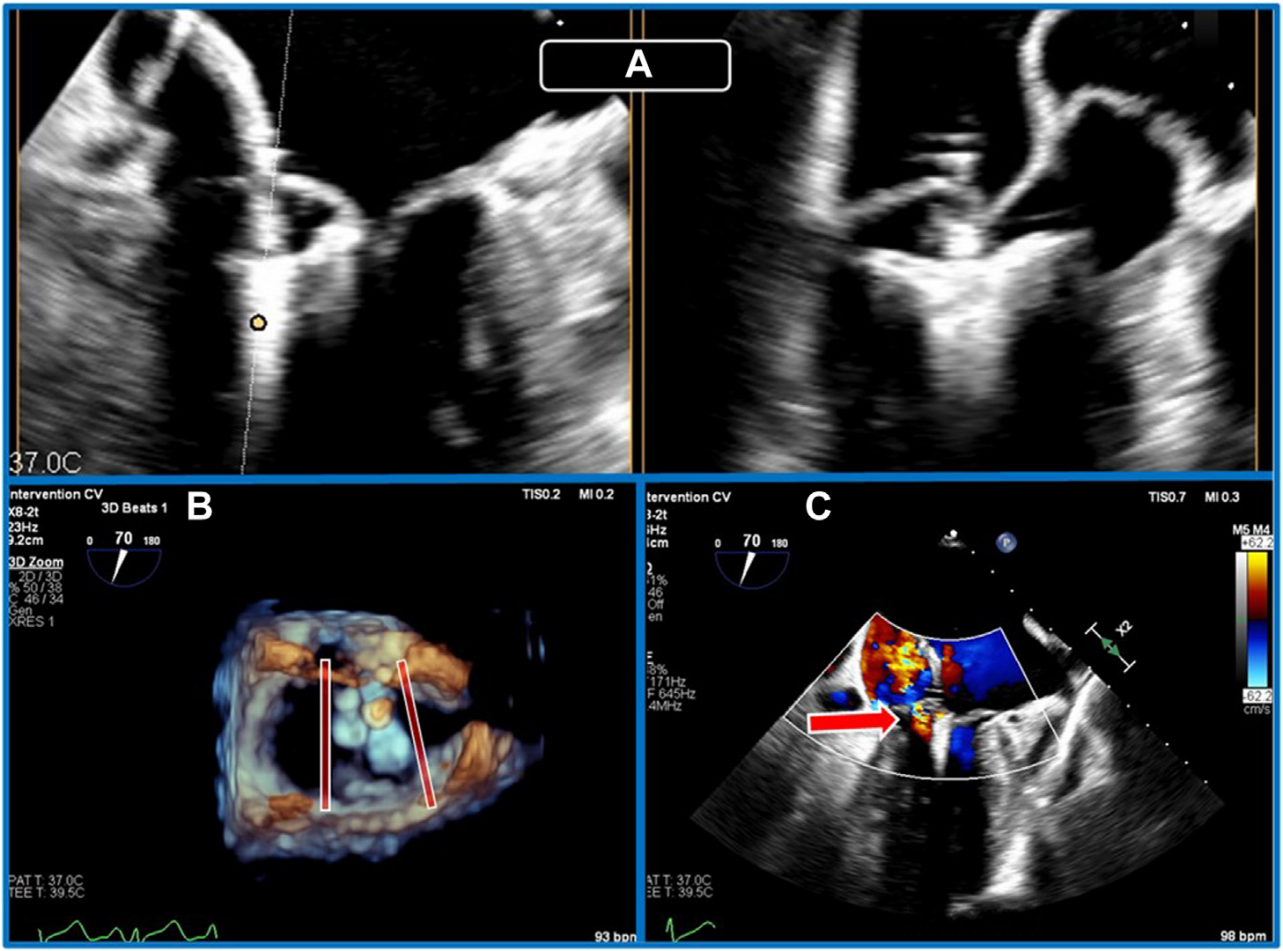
Unsuccessful first attempt at placement of a medial second device. Three-dimensional TEE, midesophageal, RT-MPR simultaneous orthogonal long-axis views (A) and short-axis volume-rendered reconstruction en face MV (B) and 2D bicommissural (70°) view with color flow Doppler (C), demonstrates optimal grasping view, near parallel alignment of the devices *(red lines)*, and residual MR *(arrow)* despite good grasp (note proximal flow acceleration despite a normal Nyquist limit).
